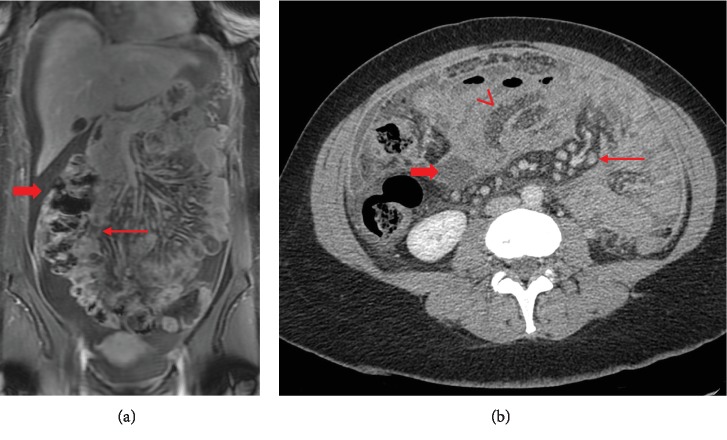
(a) Coronal fat sat T1-weighted MR image with intravenous contrast injection showing parietal thickening of the terminal ileum (long arrow) and abdominal ascites (thick arrow). (b) CT scan showing mesenteric adenopathy (long arrow), thickening of the ileum (arrowhead), and mesenteric fluid (thick arrow).
